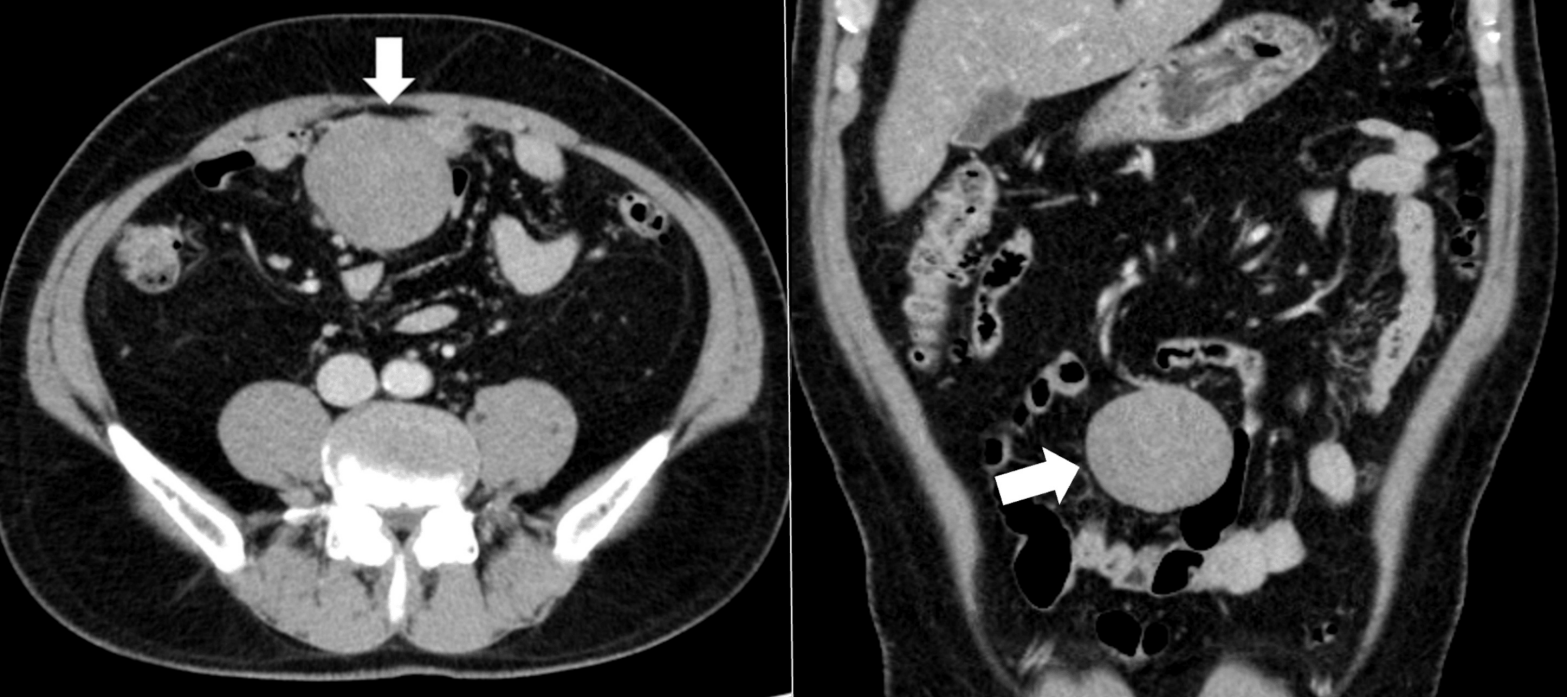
Contrast-enhanced CT scan of lesion 1 showing heterogeneous internal enhancement, measuring 6 cm in diameter (white arrows). CT, computed tomography
